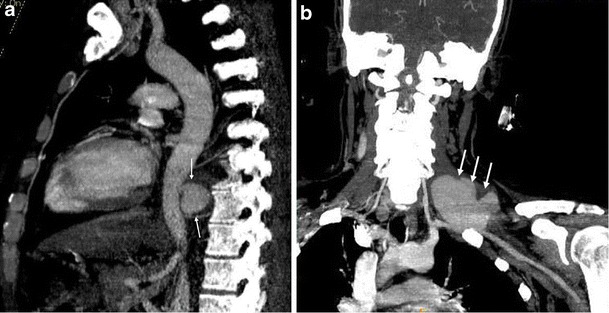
a, b A 40-year-old male with Behçet's disease. Sagittal maximum-intensity-projection CT image (a) shows a saccular aneurysm arising from the distal thoracic aorta (*arrows*). Coronal maximum-intensity-projection CT image (b) shows saccular aneurysm arising from the left subclavian artery (*white arrows*)
